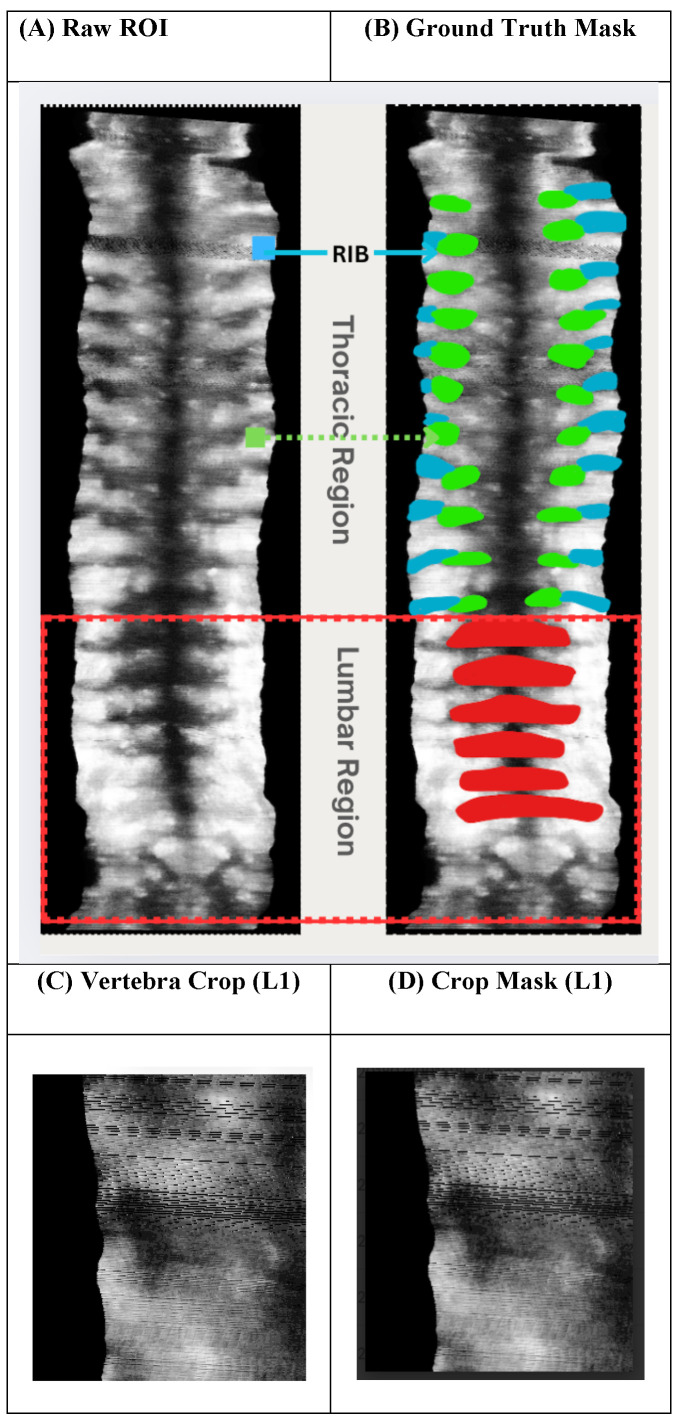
An example of a spinal ultrasound dataset and annotation strategy.
